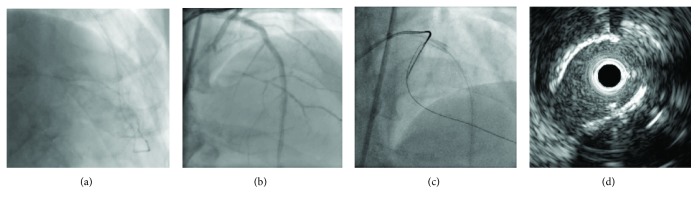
(a) Tip injection reveals the connection from the apical channel to the posterolateral branch. (b) The bidirectional angiography shows the short chronic total occlusion length. (c) The Gaia 2^nd^ directly crosses the chronic total occlusion lesion. (d) The intravascular ultrasound imaging confirms that the retrograde wire is in the true lumen.
